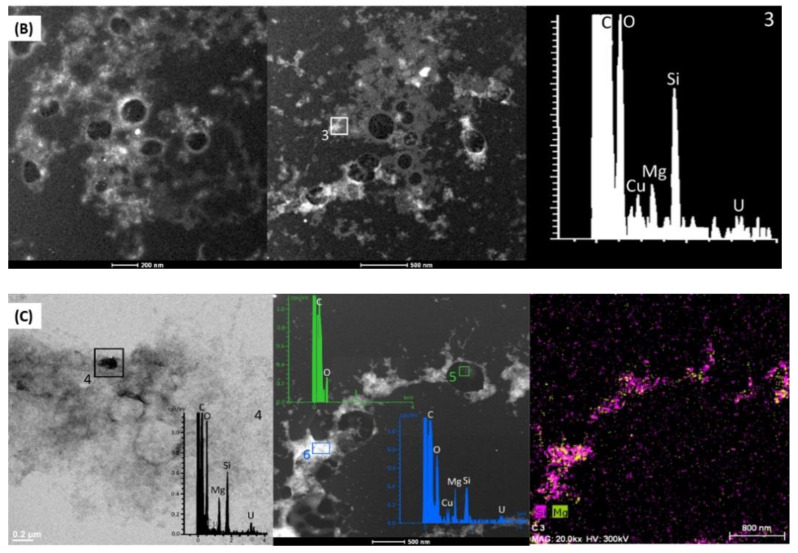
UHRTEM microphotographs, XEDS analyses, and elemental X-ray maps of MMn_L (A), MMn2_L (B), and MMn4_L (C). Scale bars = 200 nm.
